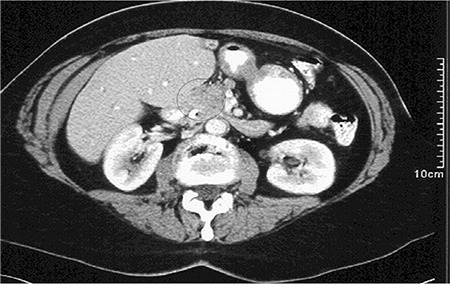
Computed tomography scan of the bile duct mass. The image shows an enhancing mass in the porta hepatis.
